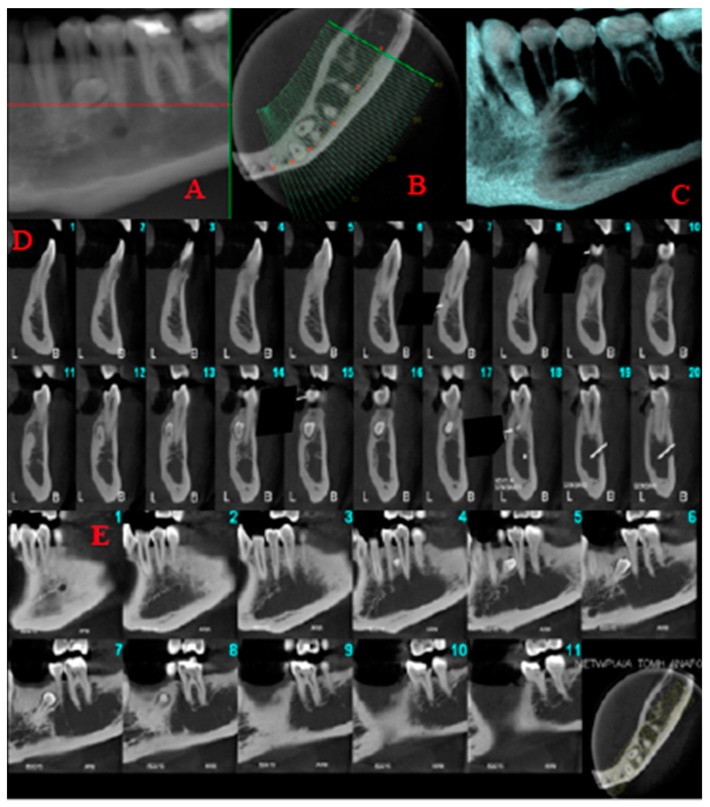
Limited field of view cone beam computed tomography images. Reformatted panoramic (A), axial (B), volumetric rendering (C) and sequential 1 mm thick/1 mm interval cross-sectional (D) and sagittal (E) cone beam computed tomography images of the left mandible demonstrating the supernumerary tooth, which is lingually located in the root of the lower left first premolar and is tangent to the latter.
